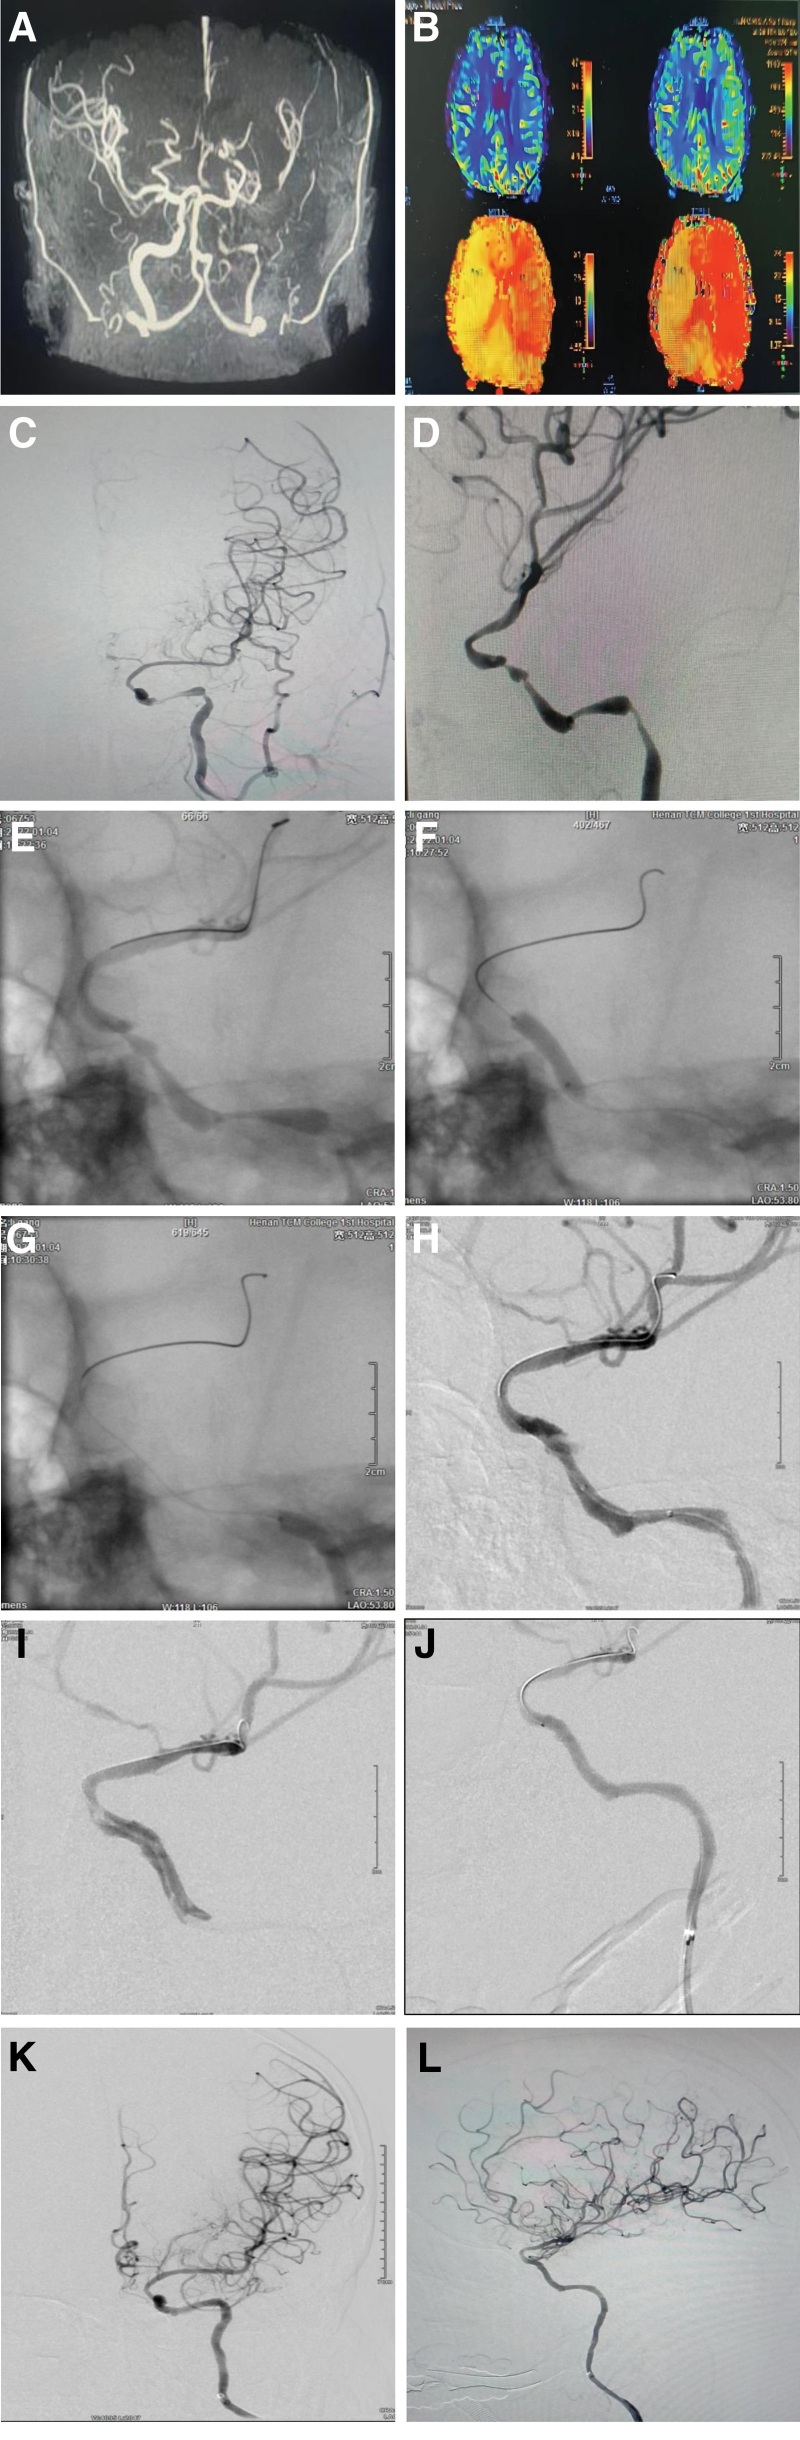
(A) MRA showed severe stenosis of the left internal carotid tandem; occlusion of the left anterior cerebral artery and severe stenosis of the left middle cerebral artery. (B) CTP suggested that the left side of the brain had ischemia-reperfusion alterations. (C, D) Left internal carotid artery with severe stenosis from C2 to C4 and poor visualization of the left anterior cerebral artery on orthopantomography and lateral angiography. (E) Intraoperative microguide placed in M3 segment. (F–H) Low-pressure balloon dilatation from C4 to C2 segments, stenosis lifted, clamping in C4 segments. (I) Stent implanted in the C4 segment with perfect apposition to the vessel wall and disappearance of the entrapment. (J) Third stent implanted in C2 segment with well-placed splice. (K, L) Postoperative internal carotid artery orthopantomography and lateral angiography with release of stenosis and significant improvement in intracranial vascular filling compared to the preoperative period.
